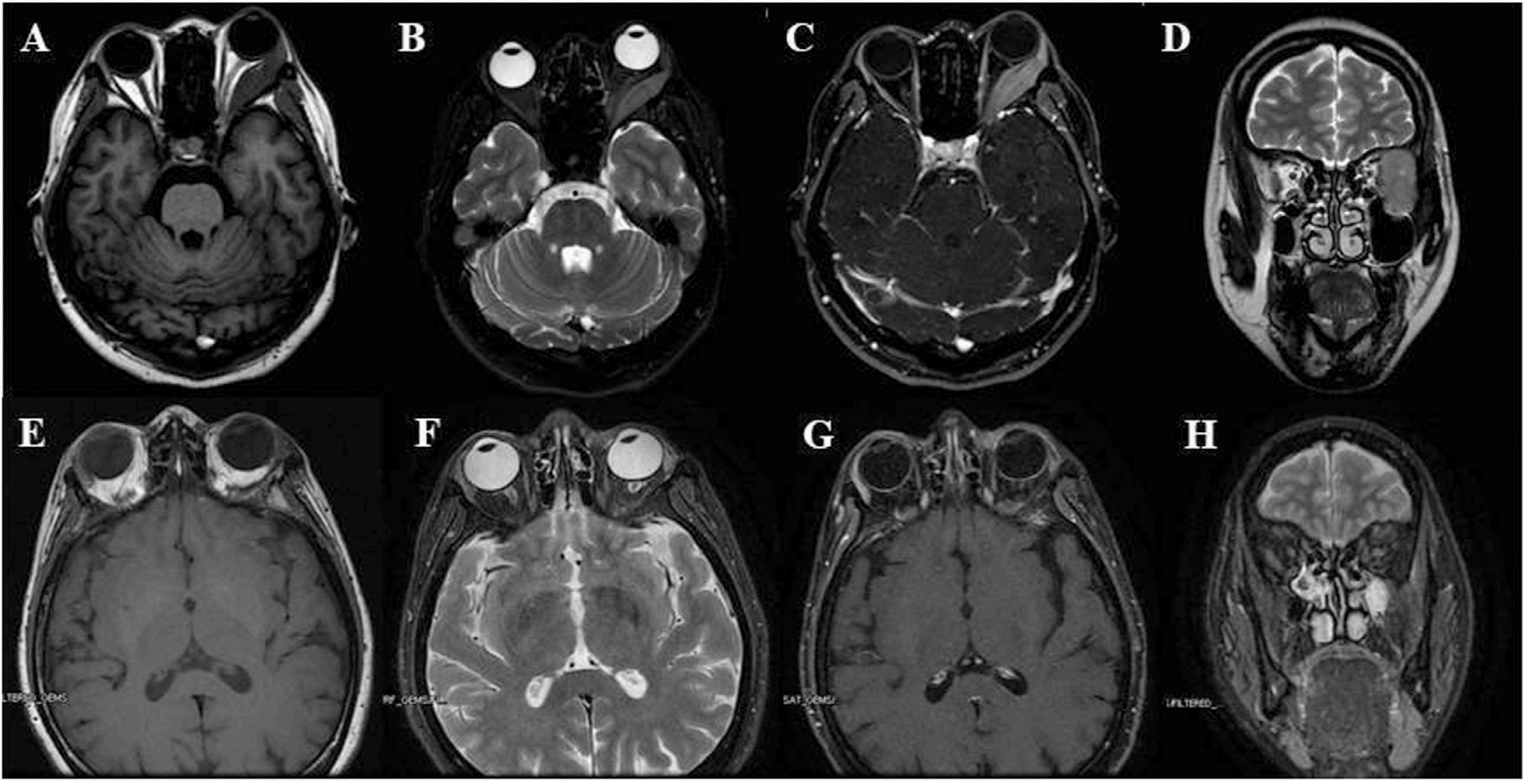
(A–D) Pre-operational orbital MRI showing T1W (A), fat-suppressed, axial T2W (B), fat-suppressed, strengthen scanning, axial T1W (C) and coronal T2W (D) images, it presented a well-defined expansive mass with low T1 and slightly high T2 signals, and pushed the lateral rectus and optic nerve; (E–H) Post-operational orbital MRI showing T1W (E), fat-suppressed, axial T2W (F), fat-suppressed, strengthen scanning, axial T1W (G) and coronal T2W (H) images: no nodule or mass was observed in left orbit.
